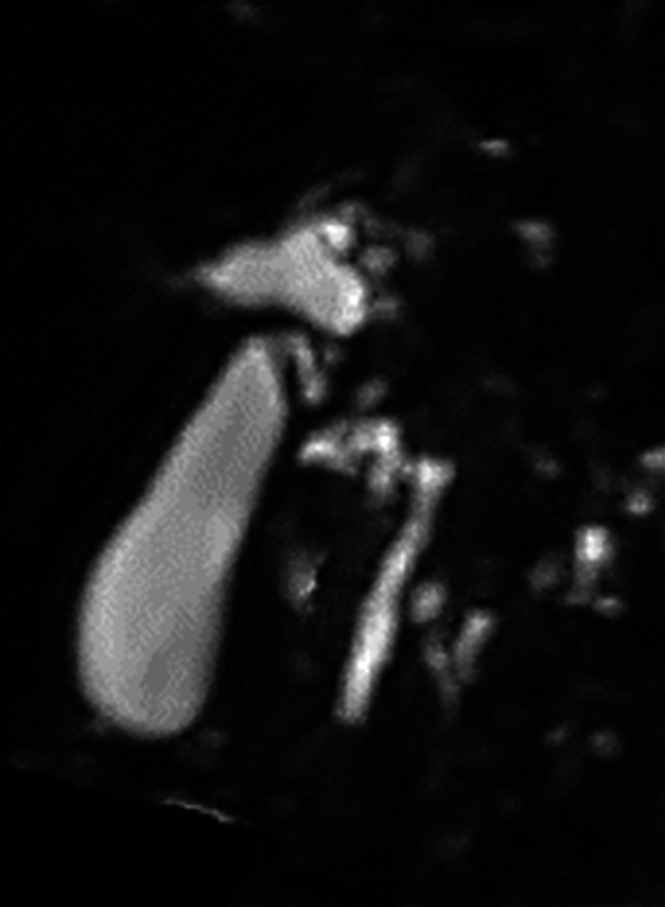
Preoperative magnetic resonance imaging (MRI) findings. MRI findings at 126 days of age revealed dilatation of the intrahepatic bile ducts, common hepatic duct, and gallbladder. The common hepatic duct ran to the left side. Dilatation of the common bile duct was not observed. It was difficult to identify the presence or absence of a pancreaticobiliary maljunction.
